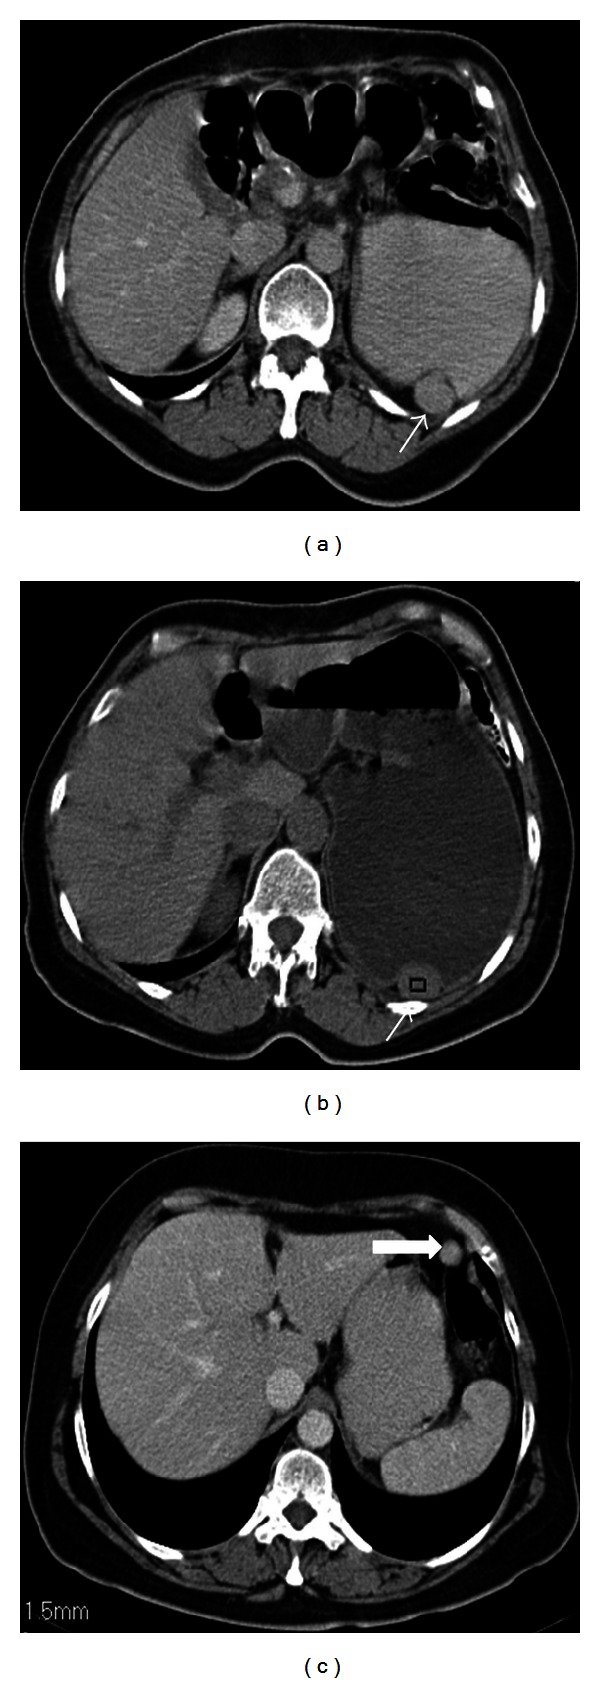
Accessory spleens can locate at the wall of stomach or bowel. Axial contrast-enhanced CT image (a) with oral contrast medium shows a nodular hyperdense lesion (arrow) indenting the posterior wall of the stomach in a 57-year-old female patient. The follow-up axial nonenhanced image (b) with oral water shows persistence of its high density indicating an accessory spleen. Axial contrast enhanced CT image (c) shows an accessory spleen located closely to the colonic wall (thick arrow).
